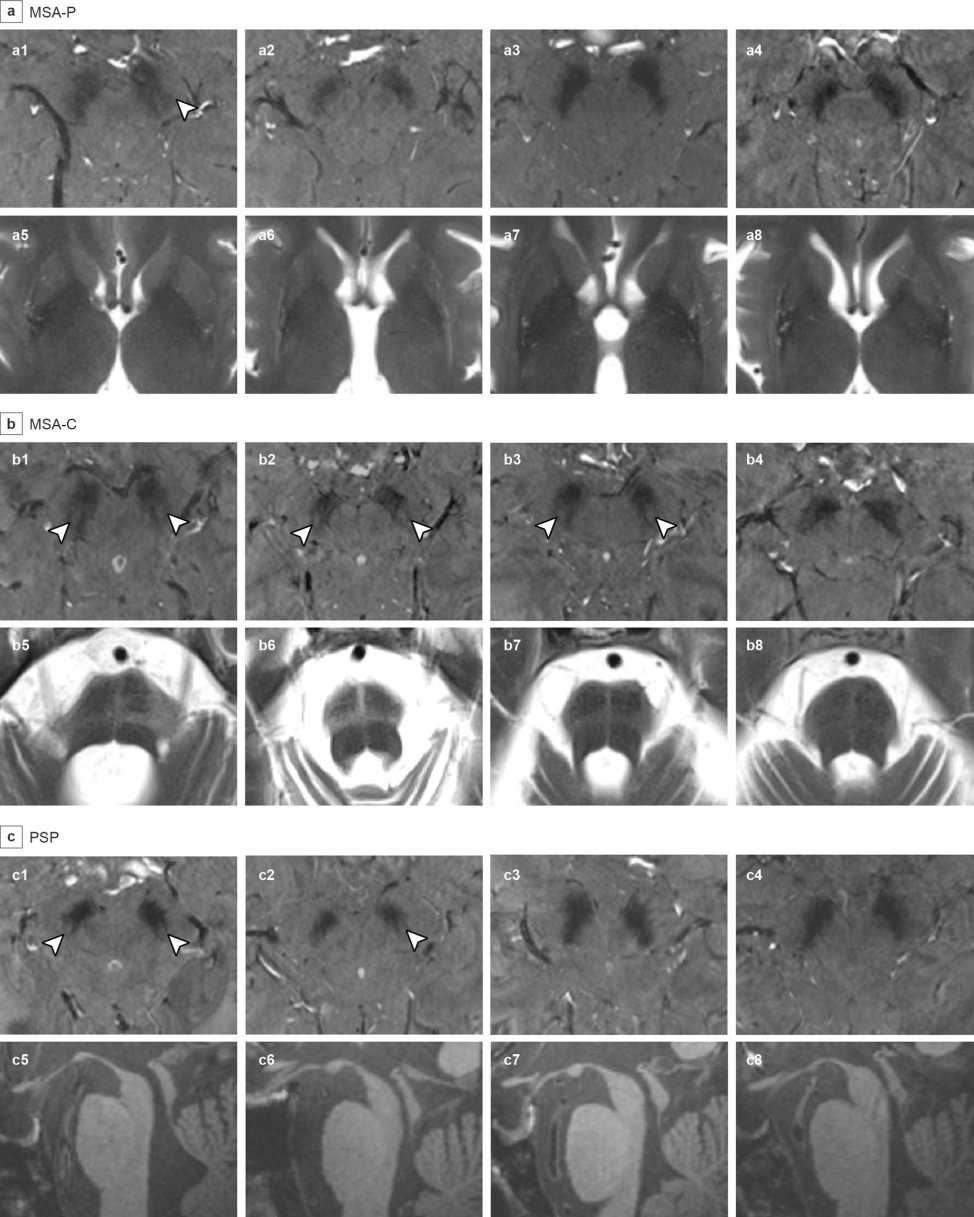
7T 3D gradient-echo T2*-weighted images at echo 2 of the substantia nigra and corresponding 3 T T1/T2-weighted images in MSA-P (a), MSA-C (b), and PSP (c). Arrowheads indicate detection of DNH. DNH was detected in a1, b1-b3, and c1-c2, and absent in a2-a4, b4, and c3-c4, indicating the heterogeneity of DNH abnormality in MSA-C. Corresponding axial T2-weighted images of the basal ganglia show atrophy in the putamen of MSA-P patients (a5–a8). Axial T2-weighted images of the pons show the hot cross bun sign in MSA-C patients (b5–b8). Sagittal T1 images reveal midbrain atrophy and the hummingbird sign in PSP patients (c5–c8). MSA, multiple system atrophy; MSA-P, MSA-parkinsonian type; MSA-C, MSA-cerebellar type; PSP, progressive supranuclear palsy; 3D, three-dimensional; DNH, dorsal nigral hyperintensity
